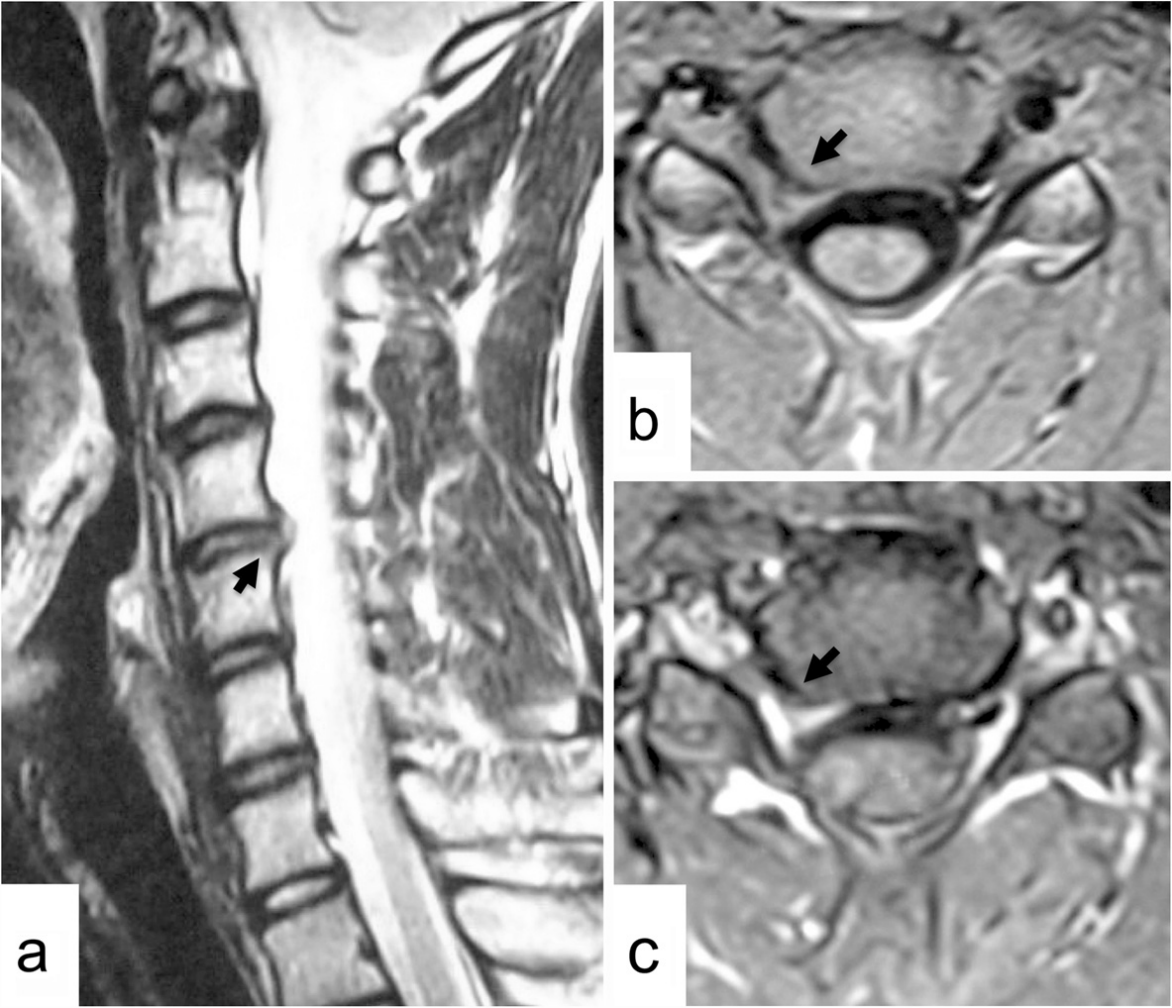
Case 2. Right parasagittal T2-weighted magnetic resonance imaging (MRI) scan of the cervical spine (a) and axial T1-weighted MRI scan at the C4-C5 level (b) showing a herniated intervertebral disc in the right C4-C5 intervertebral foramen (arrows). The herniated disc is partially enhanced after contrast medium (c) (arrow).
